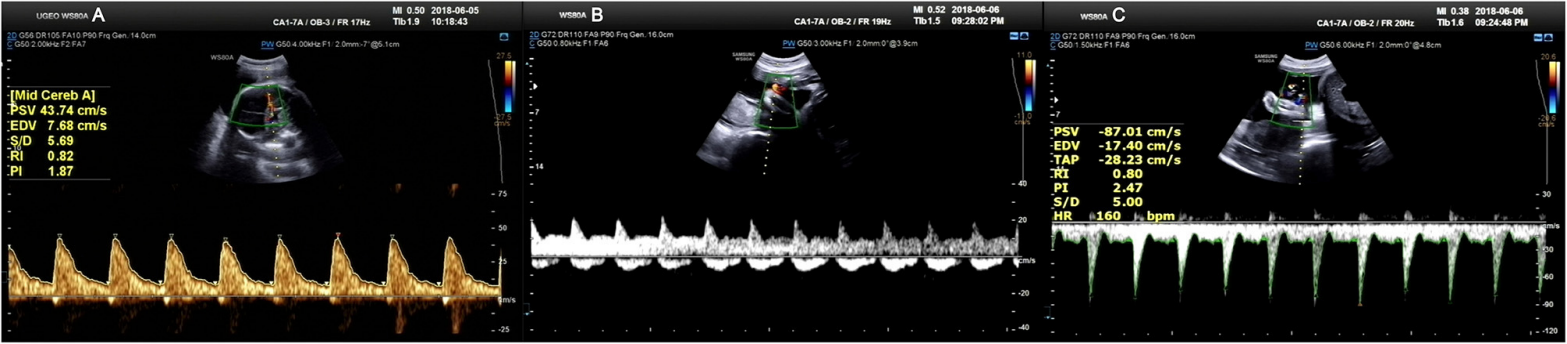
Doppler ultrasound scan at 31^+1^ weeks gestation. (A) Various blood flow indexes of the fetal middle cerebral artery. (B, C) Various blood flow indexes of fetal umbilical artery.
